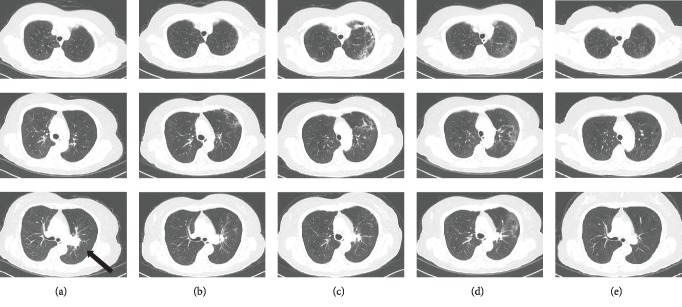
Changes in chest computed tomography images. Before treatment with nivolumab (a), at the onset of CIP (b), 1 month after the onset of CIP (c), 3 months after the onset of CIP (d), and 6 months after the onset of CIP (e). The relapsed lung tumor is indicated by the arrows. CIP: checkpoint inhibitor pneumonitis.
